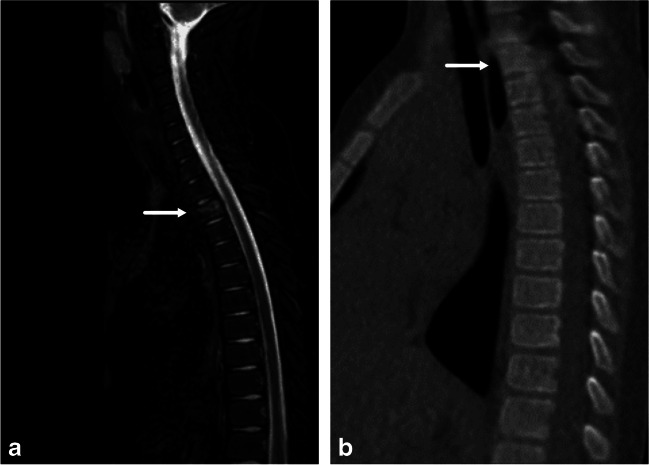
Compression fracture (*arrows*) of the third thoracic vertebra in a 9-year-old boy who fell from a swing. A low-dose computed tomography (CT) scan was performed to exclude a suspected sternal fracture. The vertebral fracture was not visible on CT scan. a Sagittal short tau inversion recovery magnetic resonance image. b Low-dose non-contrast-enhanced thoracic CT, sagittal reformat
